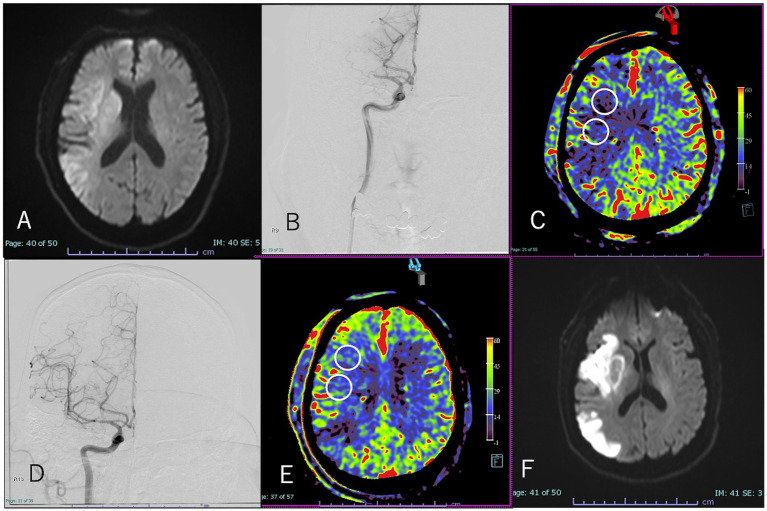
Representative case of poor parenchymal blood volume (PBV) reperfusion after endovascular thrombectomy (EVT). A 66-year-old patient presented with sudden onset of severe left hemiparesis and dysarthria. On admission, National Institutes of Health Stroke Scale (NIHSS) score was 18/42. (A) Magnetic resonance angiography (MRA) reveals occlusion of the right middle cerebral artery (MCA) and diffusion-weighted imaging (DWI) shows hyperintensity in the right MCA territory. (B) Digital subtraction angiography confirms right MCA occlusion. (C) Pre-EVT flat-panel detector computed tomography perfusion (FD-CTP) demonstrates hypoperfusion on PBV mapping in the right hemisphere (white circles; pre-PBV ratio, 0.81). (D) Complete angiographic recanalization (mTICI 3) was achieved with EVT (onset-to-recanalization time, 309 min). (E) However, post-EVT FD-CTP shows no meaningful increase in PBV in the affected region (white circles; post-PBV ratio, 1.13; ΔPBV, 1.40). (F) Follow-up DWI reveals extensive infarction in the right MCA territory.
